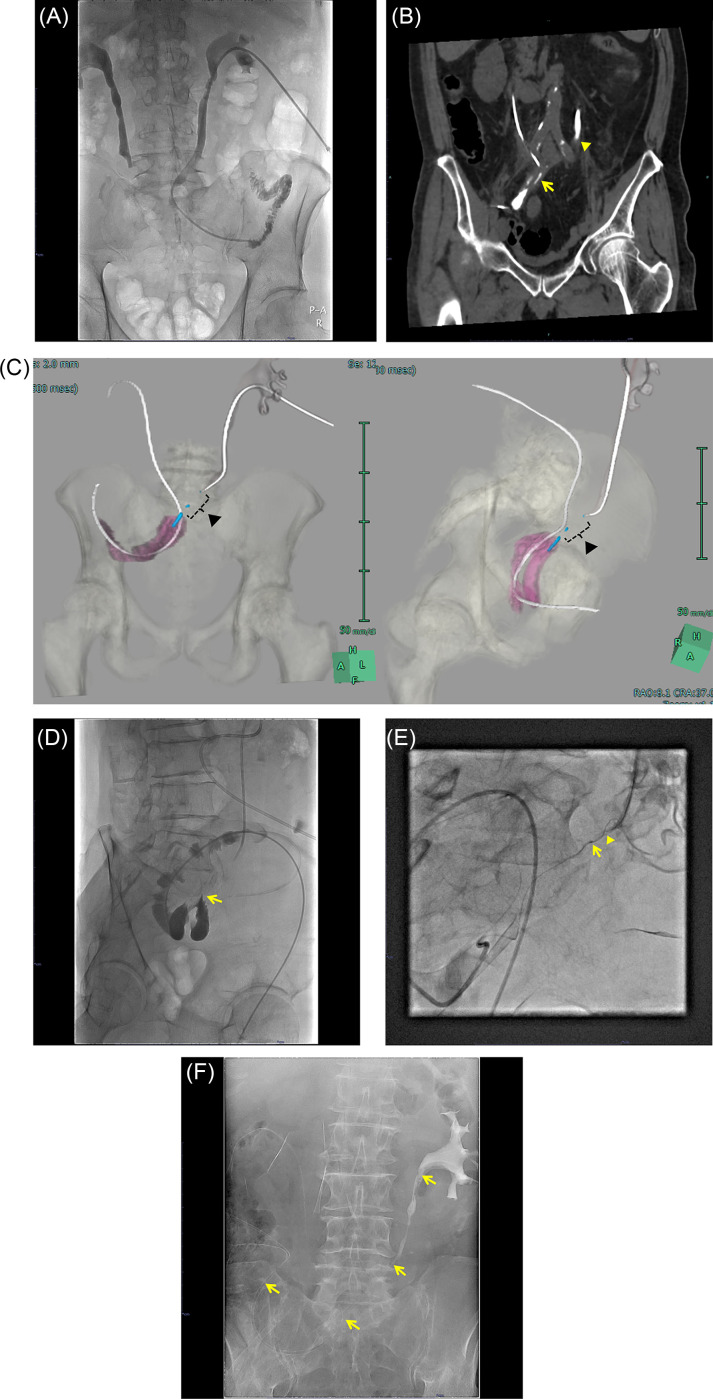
A 64-year-old man after total pelvic resection and urinary diversion with ileal conduit. He developed a postoperative urinary tract infection and a nephrostomy was constructed. (A) Bilateral nephrostomies were created owing to urinary tract infection. The right side was successfully converted to an internal-external drainage via the antegrade approach; however, the left side was failed. (B) Loopgraphy-CT image showed the anastomotic site of the left ureter and nearby ureter (arrows), and the obstructed portion of the left ureter (arrowheads). (C) The reconstructed image shows the ileal conduit (pink), ureteral anastomosis and nearby ureter (blue), and the tip of the catheter inserted through the left nephrostomy. The length of the obstructed portion was relatively long (arrowheads). (D) Based on Loopgrapgy-CT, contrast from the ileal conduit identified the left ureteral anastomosis (arrow). (E) The obstruction was broken through the rendezvous technique using microcatheters and microguidewires from both sides of the nephrostomy and ileal conduit. The microcatheter tip from ileal conduit (arrow) and penetration by microguidewire (arrowhead). (F) After establishing the pull-through route, a 6Fr internal-external drainage catheter (arrows) was successfully inserted.
